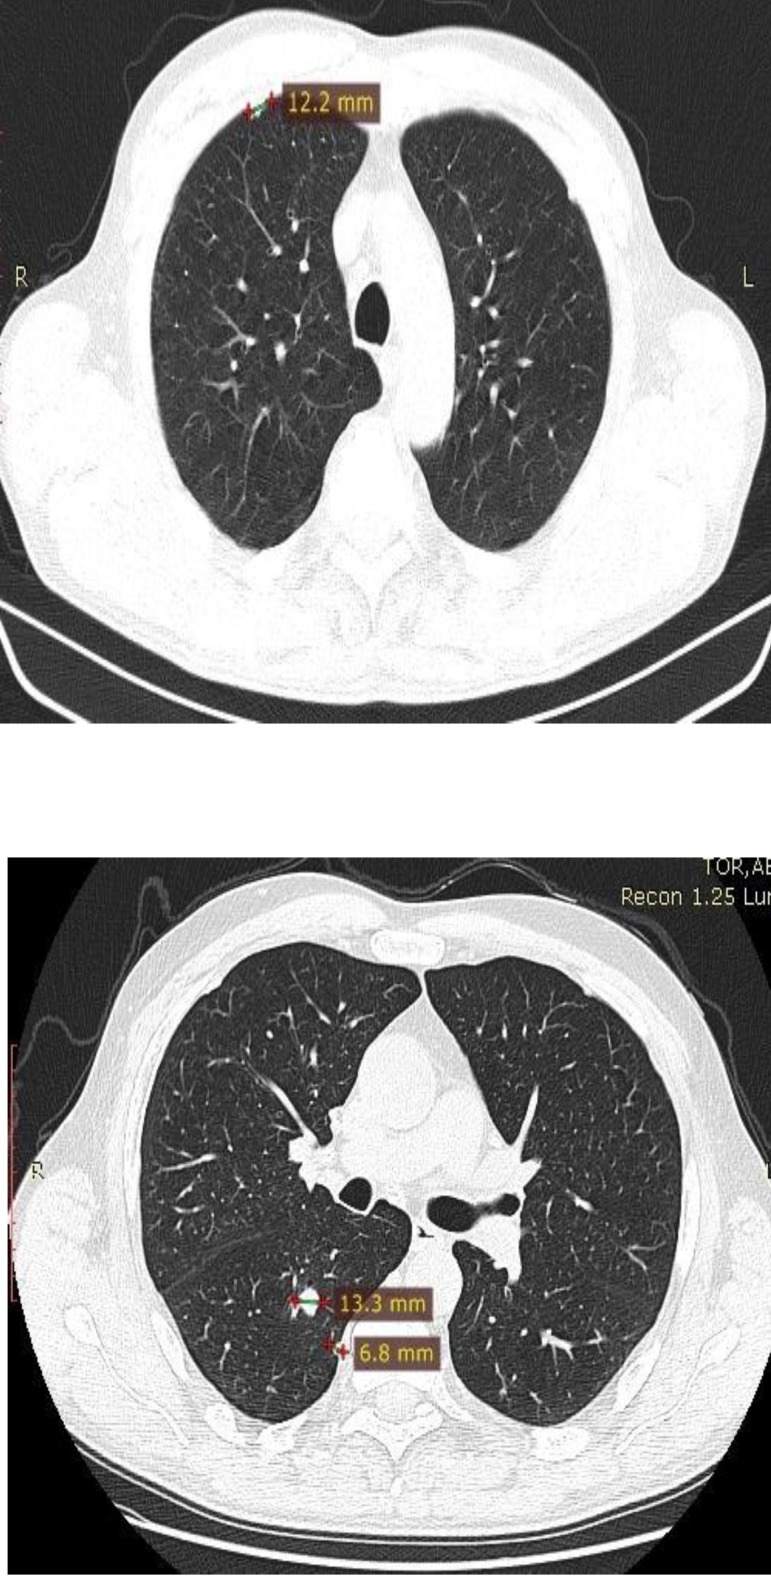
CT scan: disease progression with pulmonary metastases increased in size
